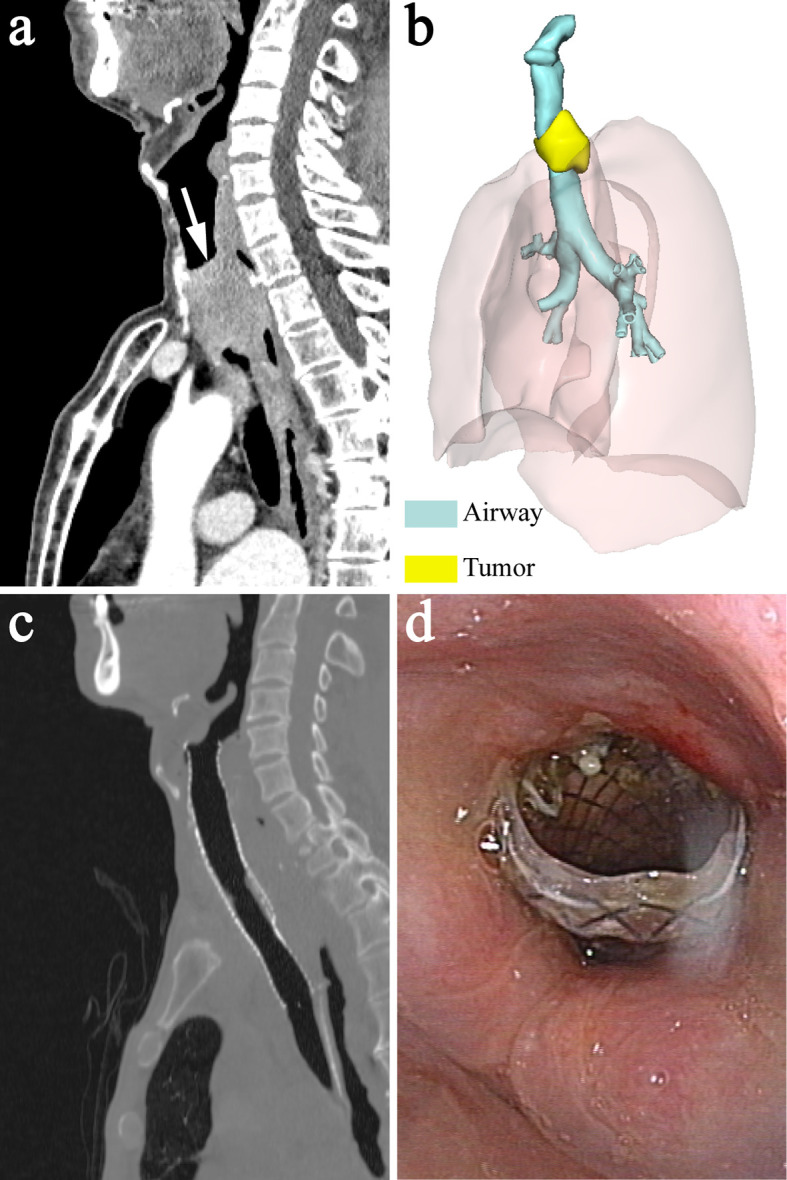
A 70-year-old man with upper tracheal stenosis caused by esophageal cancer. (A) Sagittal CT showed severe upper tracheal stenosis due to tumor compression before stenting (white arrow). (B) 3-dimensional reconstruction image showed the relationship between the stenotic upper trachea and surrounding tumor. (C) Sagittal CT showed that airway patency was restored 1 week after stenting and the proximal end of the stent was located slightly above the vocal cord and below the epiglottis. (D) Laryngoscopy 1 week after stenting showed that the proximal end of the stent was above the vocal cord, below the epiglottis and almost at the level of the arytenoid cartilages.
